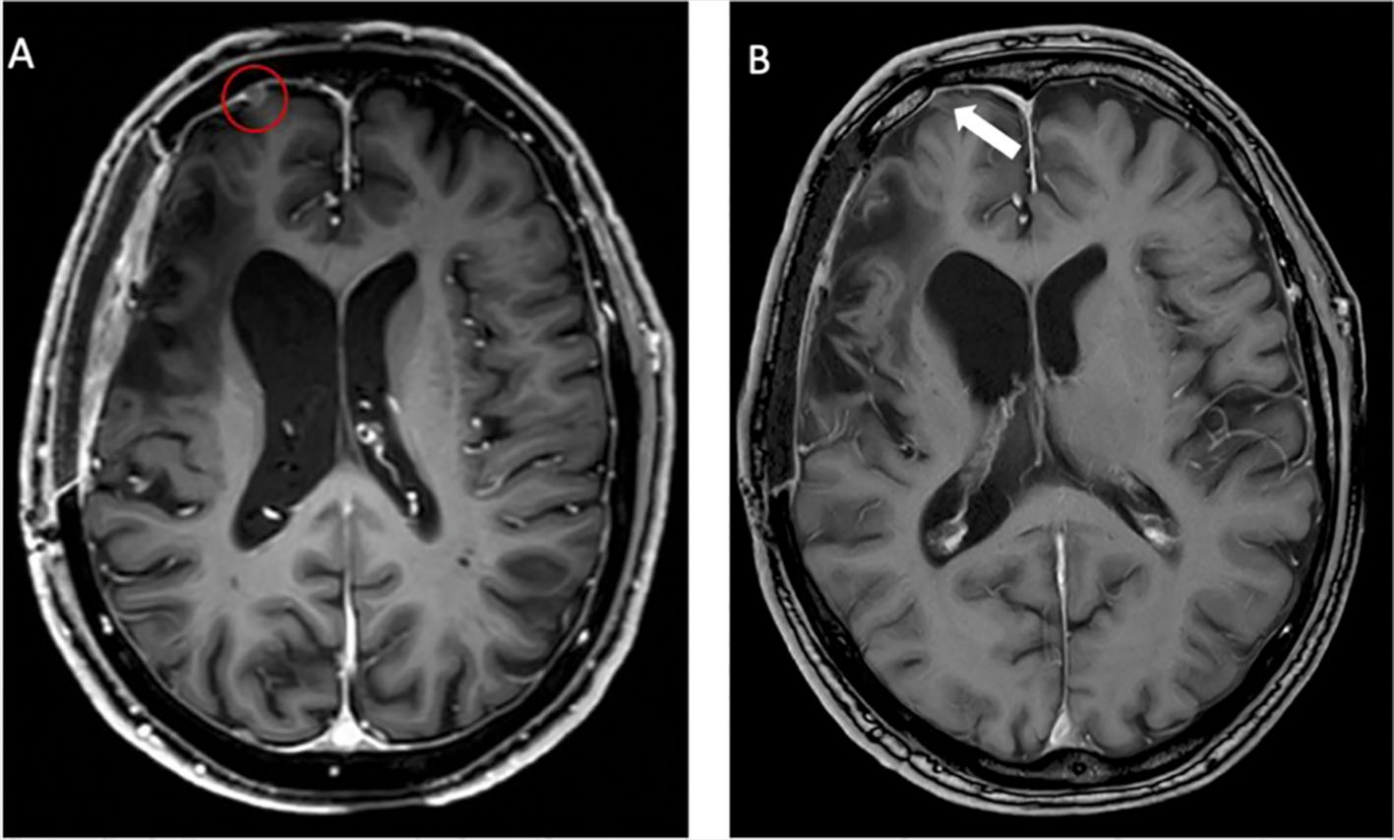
Follow-up MRI after 10 weeks (A) showing a progressive lesion (red circle) compared to the baseline MRI (B). At initial evaluation, only a small dural alteration was observed (white arrow). In contrast, PET imaging detected considerably increased somatostatin receptor (SSTR) expression as assessed by ^68^Ga-DOTATATE uptake.
